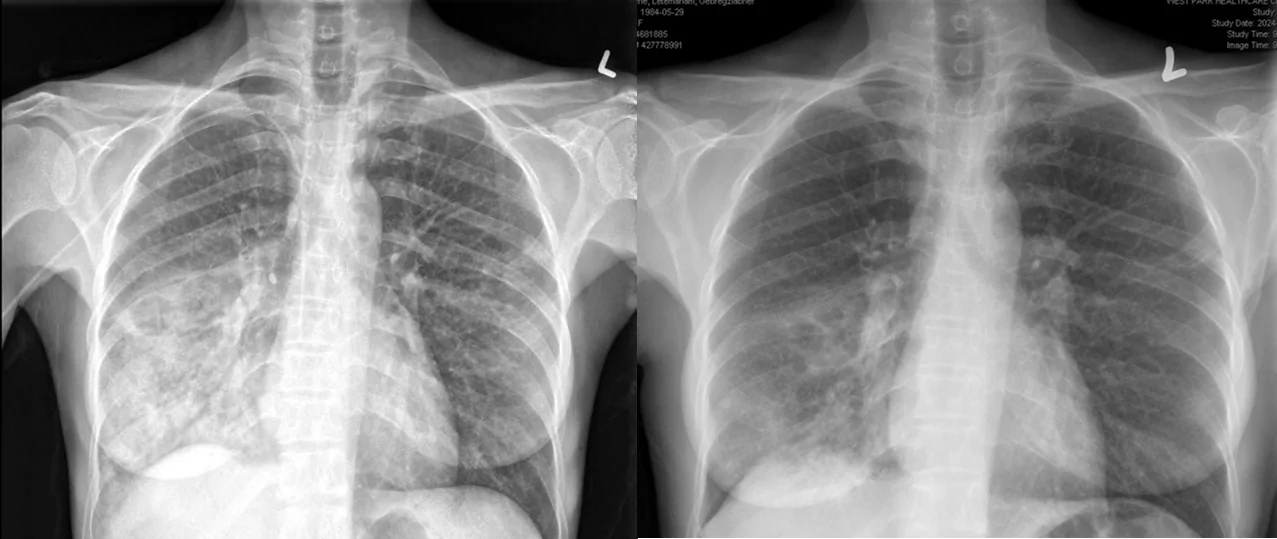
Serial chest radiographs demonstrating radiographic progression and residual disease following treatment of multidrug-resistant tuberculosis. (Left) Chest radiograph obtained at diagnosis (April 2025) demonstrating consolidation predominantly involving the right lower lobe with extension into the right middle lobe. (Right) Follow-up chest radiograph (February 2024) showing persistent right lower lobe airspace opacities with areas of lucency suggestive of evolving bronchiectasis.
